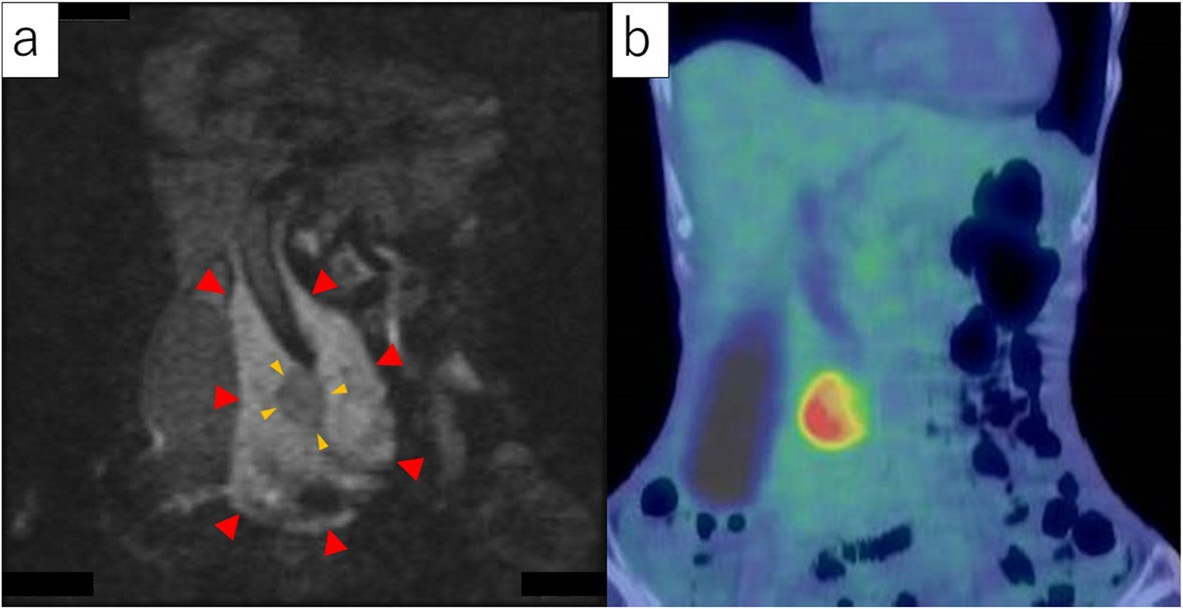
a Diffusion-weighted images of MRI. The peripheral part had a very high signal, while the central part had a relatively lower signal. b Positron emission tomography. A strong accumulation of fluorodeoxyglucose was seen in the central part, suggesting a high-grade malignant tumor
